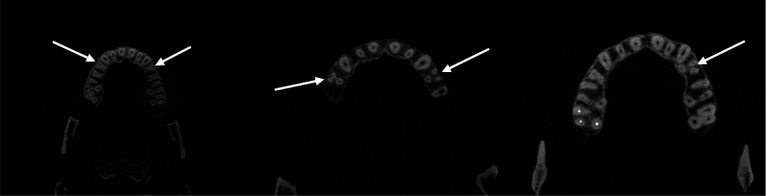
CBCT (Cone-Beam Computed Tomography) in the axial plane revealed single-rooted maxillary first and second premolars (arrows indicate the examined teeth)
